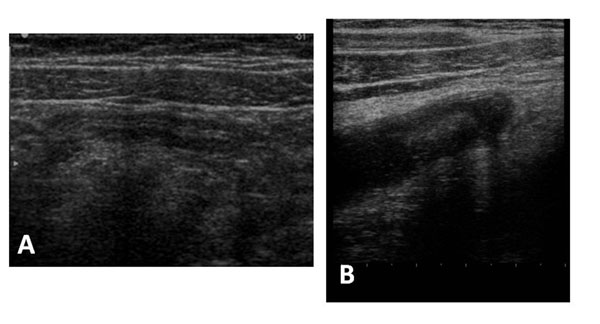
Ultrasound features of appendicitis. On longitudinal images, the inflamed, non perforated appendix appears as a fluid-filled, uncompressible, blind-ending tubular structure. The maximal appendix diameter, from outside wall to outside wall, is greater than 6 mm. (a) In early non perforated appendicitis, on the longitudinal images an inner echogenic lining representing sub-mucosa can be identified.(b)
